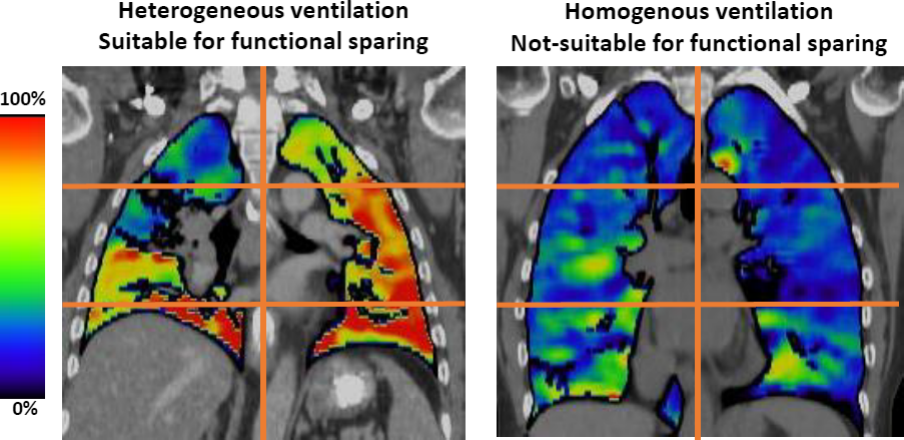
A 4DCT‐ventilation image overlaid with a standard CT is shown for two patients. An example of a patient with homogenous ventilation and a patient with heterogeneous ventilation are shown. The lines demonstrate the concept of dividing the lungs into lung thirds for evaluation.
